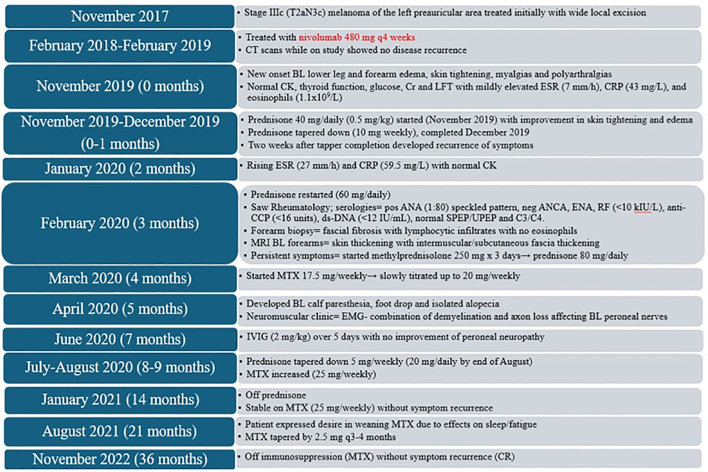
Treatment course for case 2. Timeline in months from onset of eosinophilic fasciitis. q, every; BL, bilateral; CT, computer tomography; CK, creatine kinase; Cr, creatinine; LFT, liver function test; ESR, erythrocyte sedimentation rate; CRP, C-reactive protein; ANA, anti-nuclear antibody; ANCA, antineutrophil cytoplasmic antibody; ENA, extractable nuclear antigen antibodies; RF, rheumatoid factor anti-CCP: anti-citrullinated protein antibodies; ds-DNA, double-stranded DNA, SPEP/UPEP, serum/urine protein electrophoresis; C3/C4, complement 3/4; MRI, magnetic resonance imaging; MTX, methotrexate; EMG, electromyography; IVIG, intravenous immunoglobulin; CR, complete remission.
